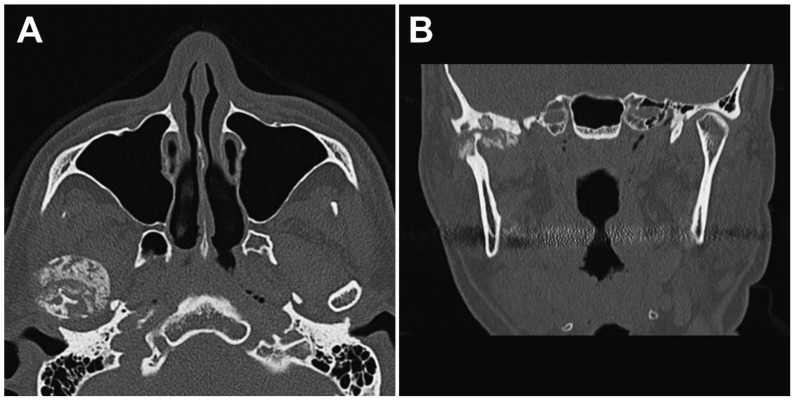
Preoperative computed tomography (CT) images. (A) Axial CT images showing the intra-articular localized, non-corticated, and ill-marginated calcified lesion that abuts the articular surface of the glenoid fossa around the right mandibular condyle. (B) Coronal CT images of the right temporomandibular joint revealed resorption of part of the mandibular condyle and cranial base.
